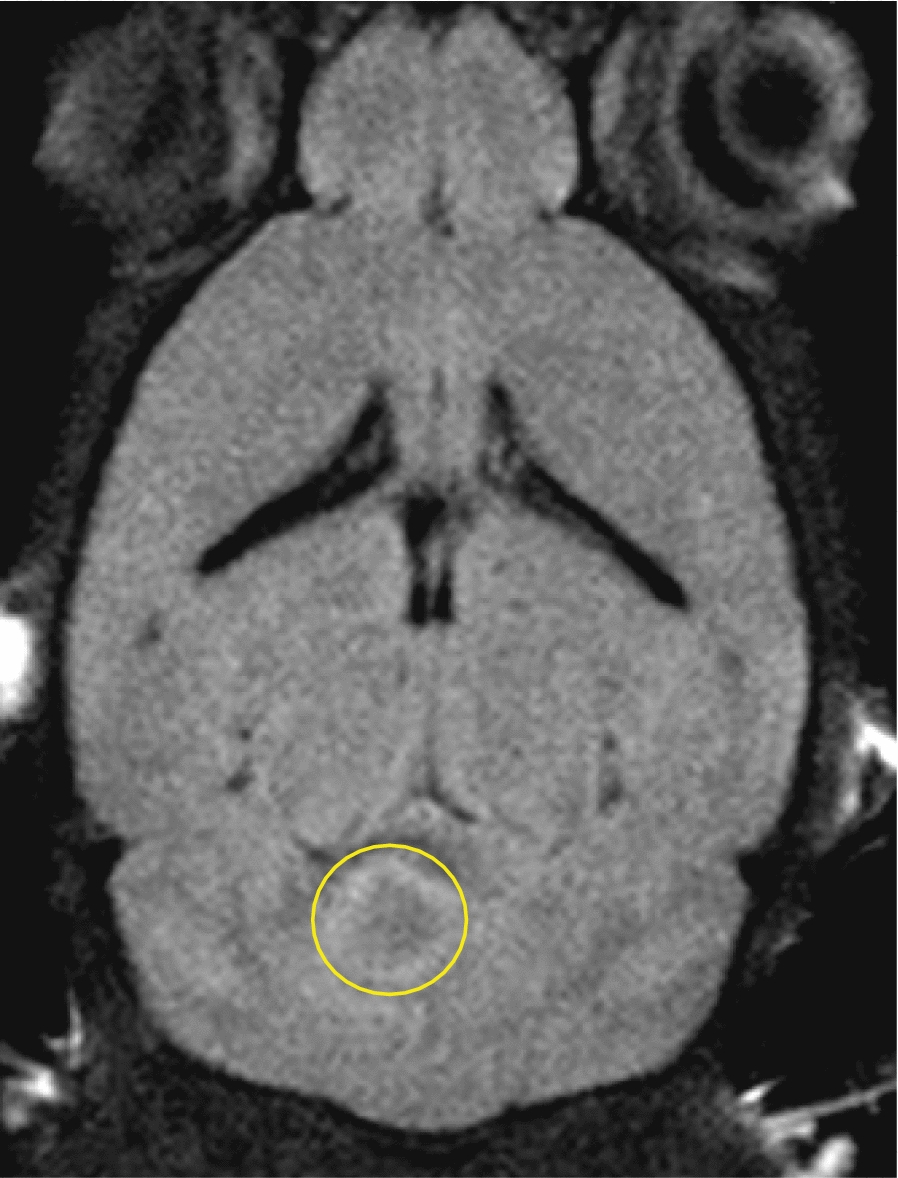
Fluid attenuated inversion recovery (FLAIR) imaging detects DIPG. FLAIR on day 14 after surgical implantation. This image identifies successful tumor engraftment
